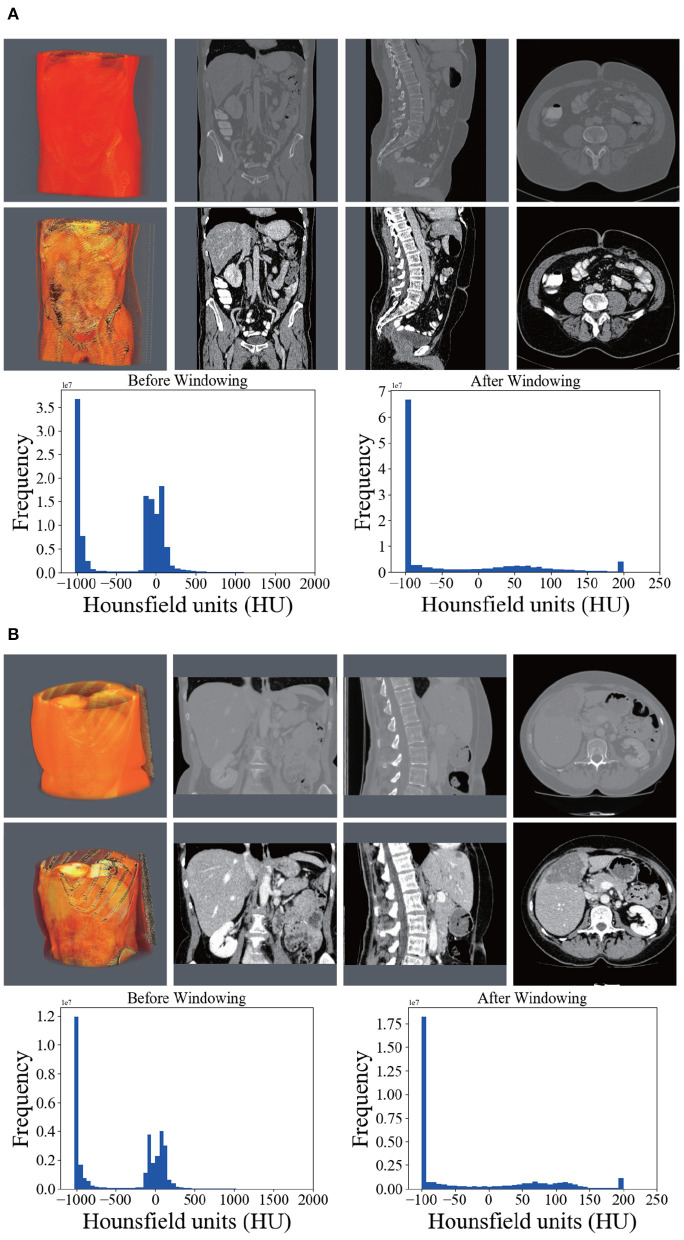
Comparison between the raw CT scans (first row), windowed (second row) scans, and histograms of HU (third row) before and after windowing. (A) Shows the comparison on LiTS. (B) Shows the comparison on 3DIRCADb.
